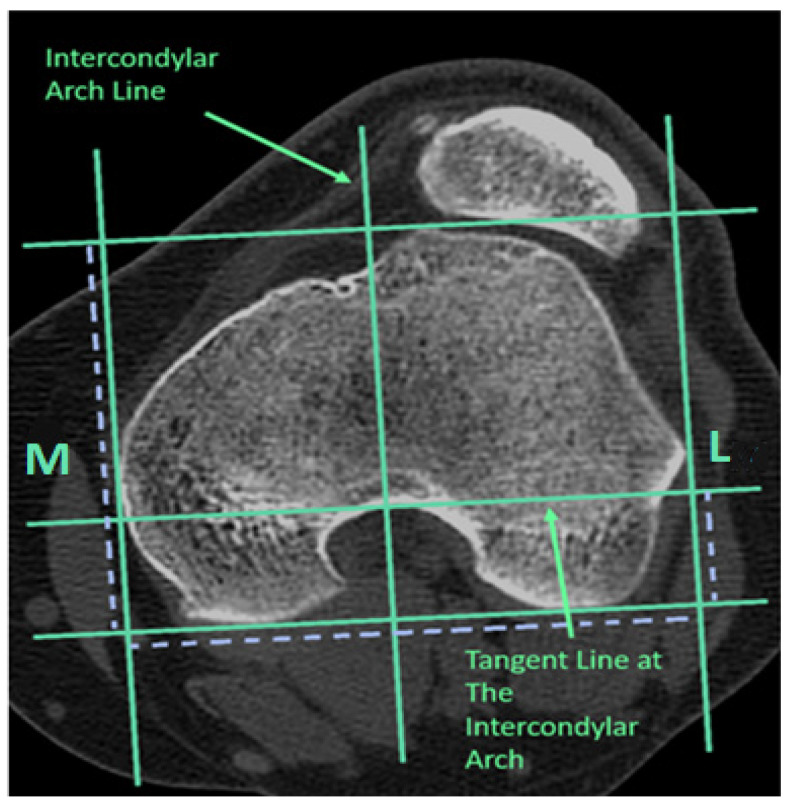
On the CT scan, the tangent line of the posterior arc, the bony landmark at the arc level, and the line of the intercondylar arc were identified (left knee). L, lateral; M, medial.
